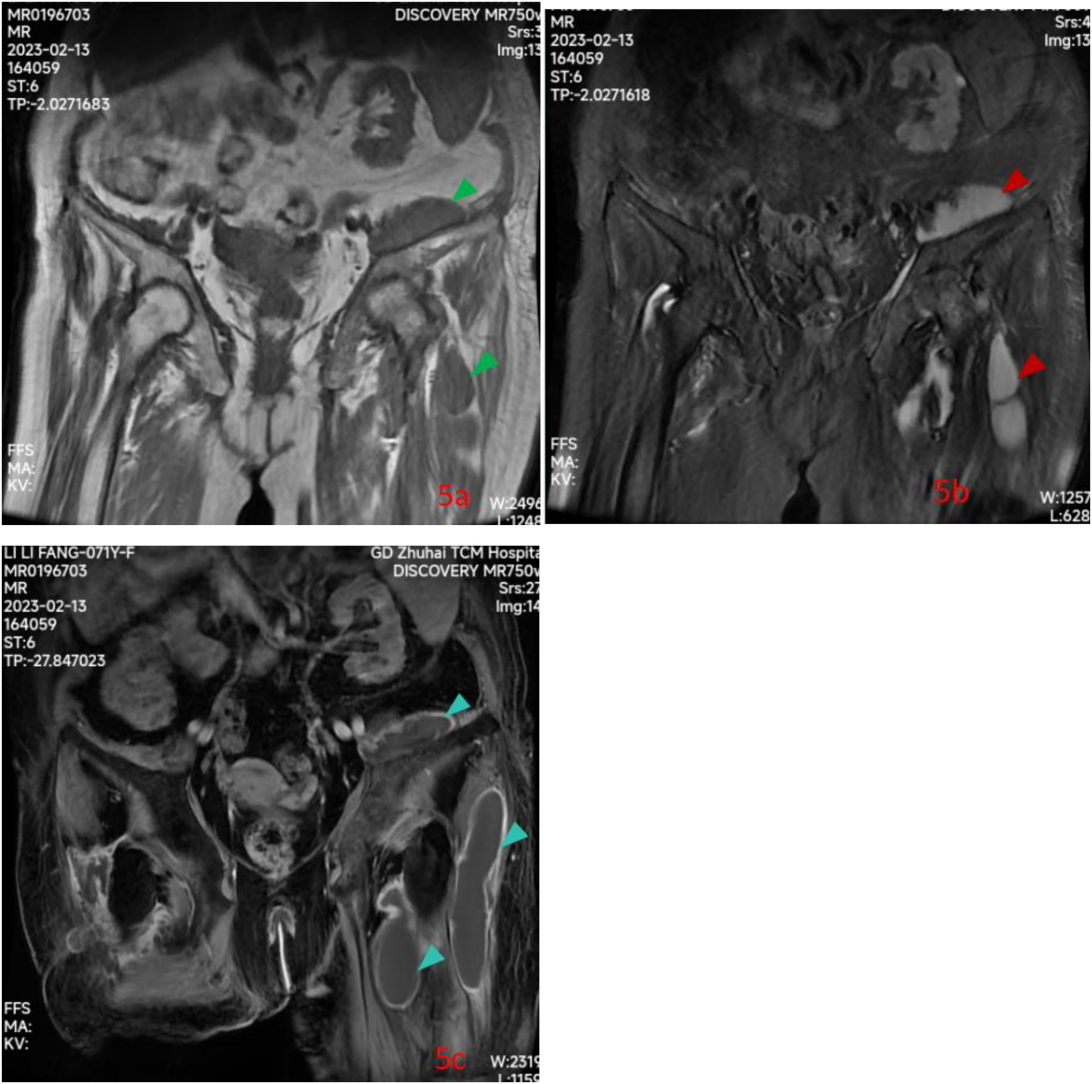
Pelvic contrast-enhanced MRI 10 days after admission showed the cystic lesions were low signal intensity (green triangle) on spin echo T1-weighted images (a), high signal intensity (red triangle) on fat-suppressed fast spin echo proton-density-weighted images (b) along the left psoas and iliacus muscles, around left hip; A large hyperdense cystic lesion (royal blue triangle) extending along the iliopsoas muscle and around left hip on enhanced scan images (c).
